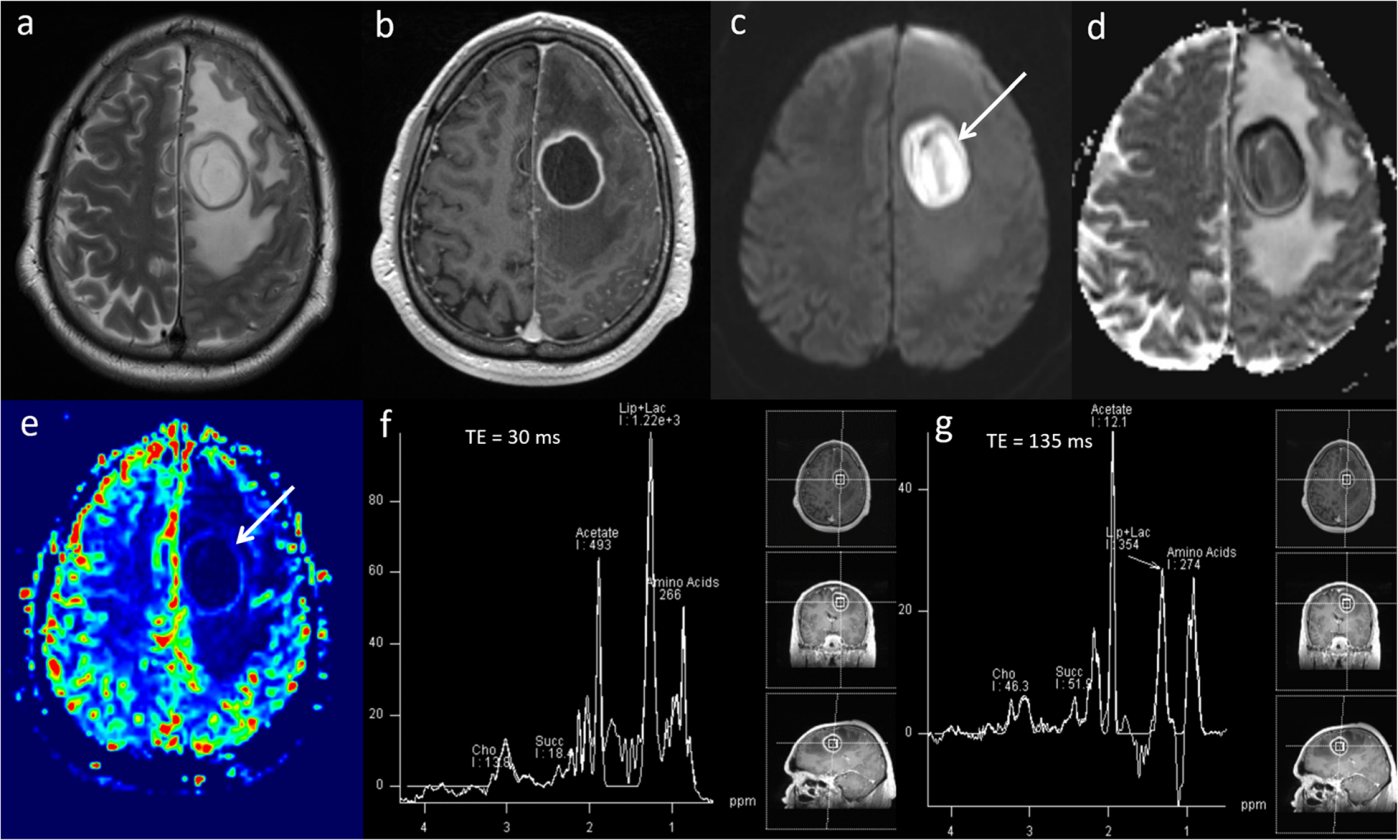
Abscess. Conventional MRI: a, b T2W and post-contrast T1W sequences demonstrate a ring-enhancing mass lesion in the left frontal lobe with surrounding oedema. c, d DWI and ADC sequences show low ADC (600 × 10^−6^ mm^2^ s^−1^, arrow) throughout the lesion. e PWI demonstrates significantly lower perfusion (arrow) than the contralateral white matter. f, g MRS shows high lipid as well as the presence of amino acid (0.9–1.0 ppm), acetate (1.92 ppm) and succinate peaks (2.42 ppm). These characteristic MRS findings in combination with the very low ADC and low perfusion are diagnostic of abscess. Diagnosis was confirmed on aspiration which revealed colonies of gram-positive cocci
